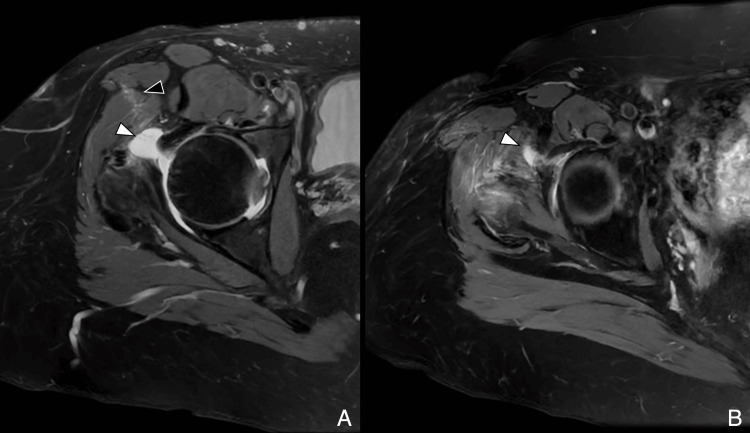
Outside-in arthroscopy (early postoperative MRI) Axial PDFS images (A) at the femoral head level and (B) above the greater trochanter show a trans-gluteal portal with TFL penetration (black arrow) and capsular lobulation at the suture plane (white arrows). Anterolateral portal path varies due to fluoroscopic placement. Image Credit: Juan Pablo Muñoz, corresponding author PDFS: proton density fat-saturated; TFL: tensor fasciae latae
